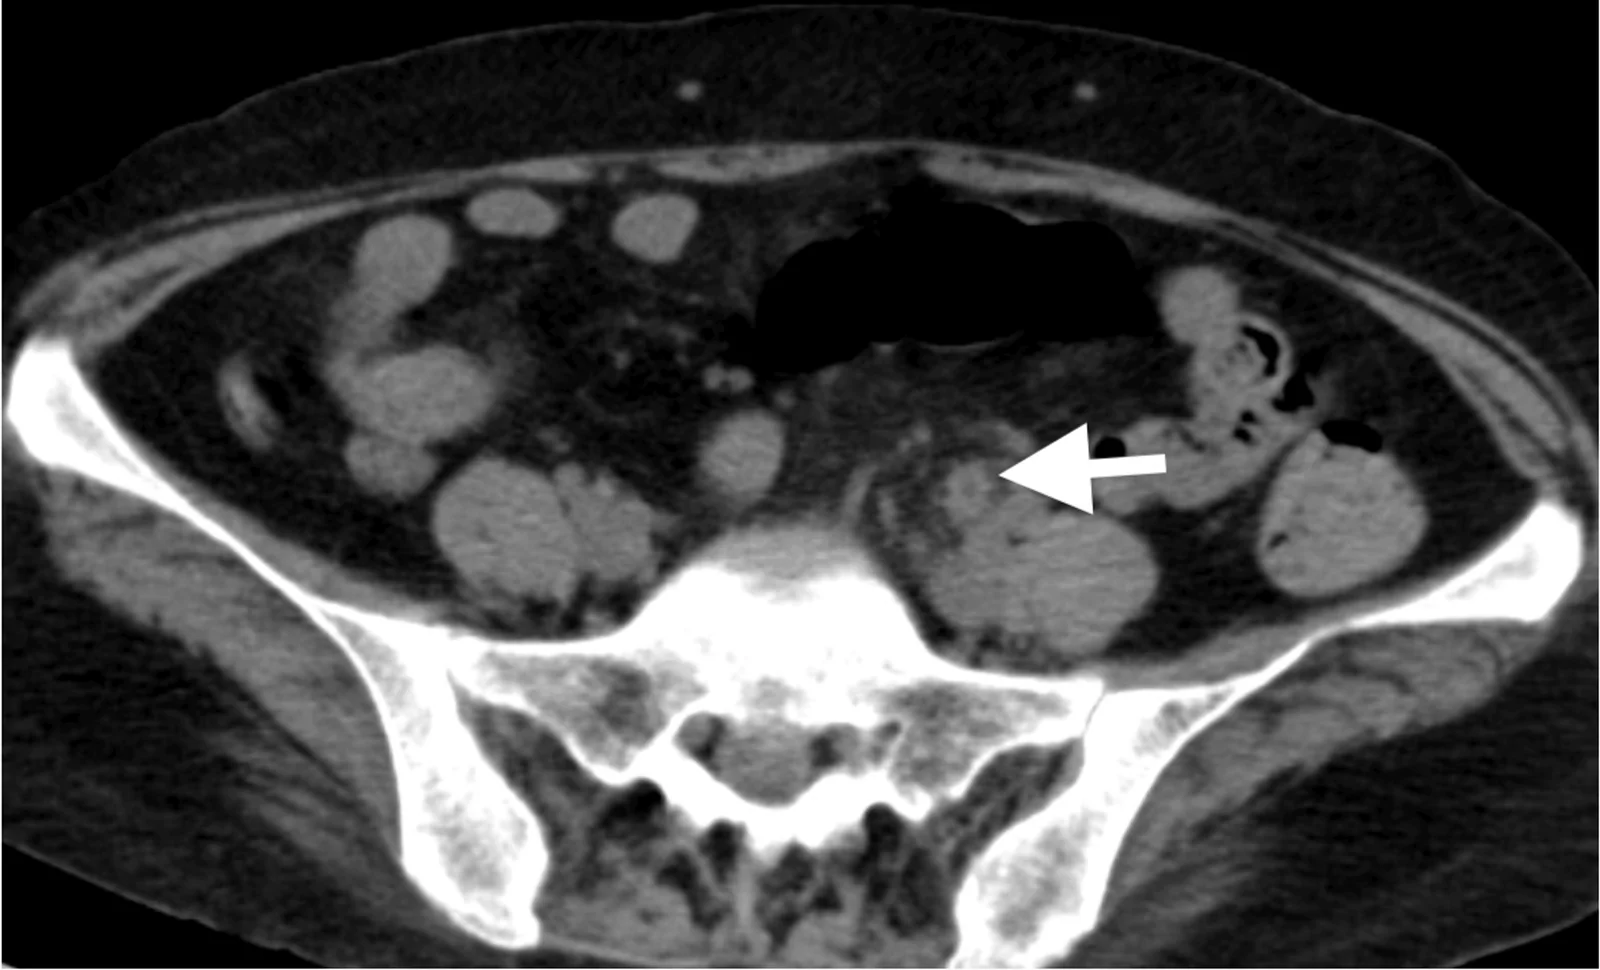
Axial non-contrast CT image of the abdomen showing a left ureteric stricture with adjacent fat stranding suggestive of inflammation (arrow). The image shows thickening of the left ureteric wall with partial luminal narrowing and adjacent periureteric fat stranding, suggesting the possibility of an inflammatory left ureteric stricture. The strictured segment measures 1.2 cm in craniocaudal length and is located at the level of the S1 vertebra. No ureteric mass lesion or calculus is seen.
